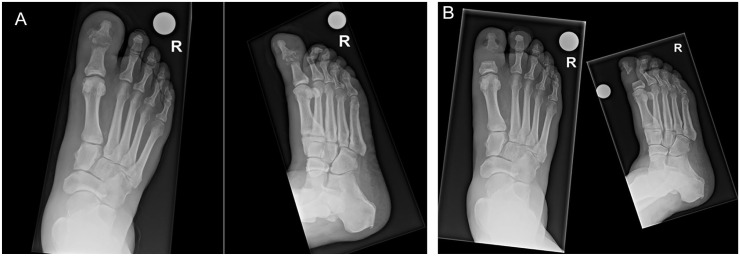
Dorsal-plantar and oblique radiographs of a 69-year-old male patient with osteomyelitis of the first interphalangeal joint (A) before and (B) after conservative surgery.
